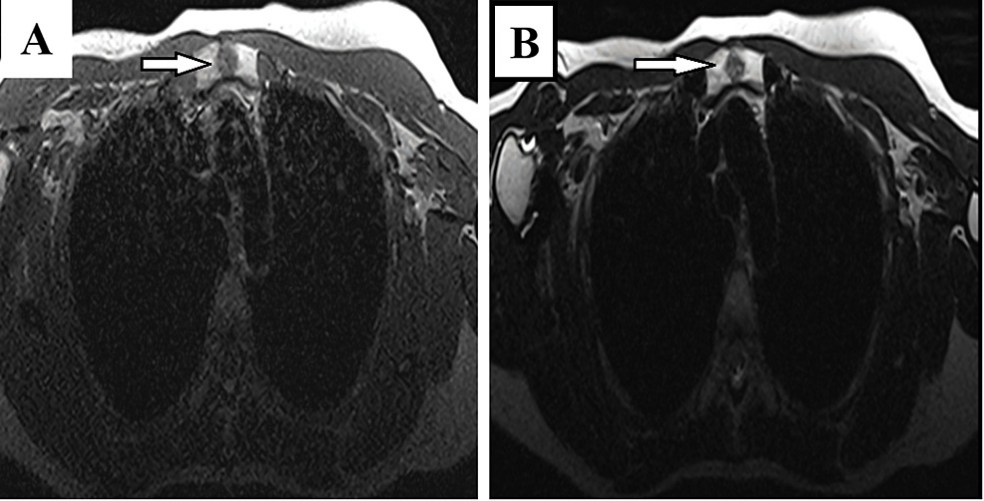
MRI imaging demonstrating the lesion in the manubrium sterni (white arrow). (A) Axial T1-weighted image reveals an intermediate signal intensity resembling muscles. (B) Axial T2-weighted image displays a heterogeneous lesion with mild hyperintensity.
